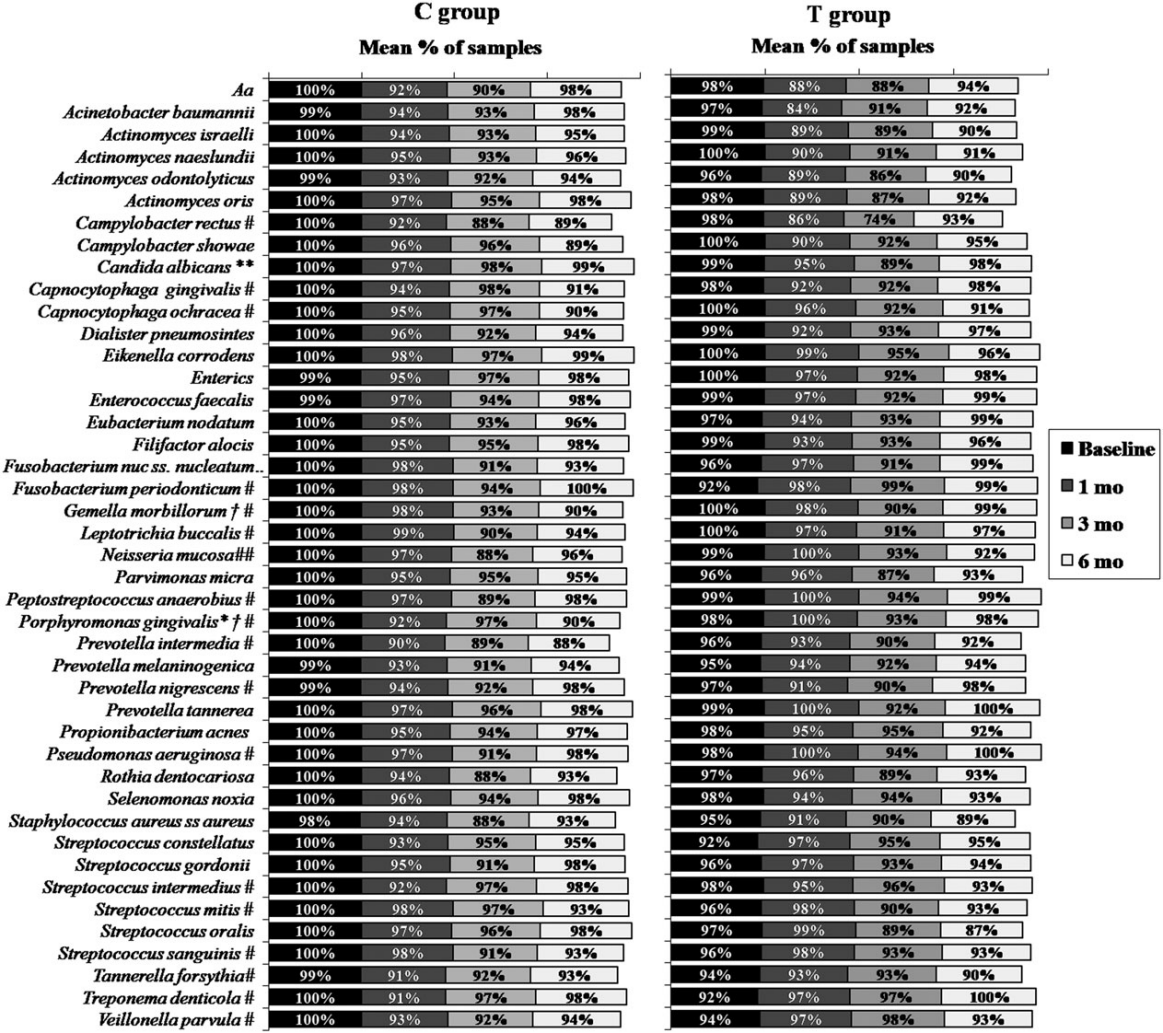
Mean frequency (%) of microbial species in subgingival plaque samples from both therapeutic groups at baseline and different posttherapy time points. C group = full‐mouth ultrasonic debridement + distilled water rinsing; T group = full‐mouth ultrasonic debridement + 0.1% sodium hypochlorite rinsing. *Aa* = *Aggregatibacteractinomycetemcomitans*. * refers to significant differences between groups at 1 month,  at 3 months, and † at 6 months (*p* < .05, Mann–Whitney test). # refers to significant changes over time within the C group, and ## within the T group (*p* < .05, Friedman test)
